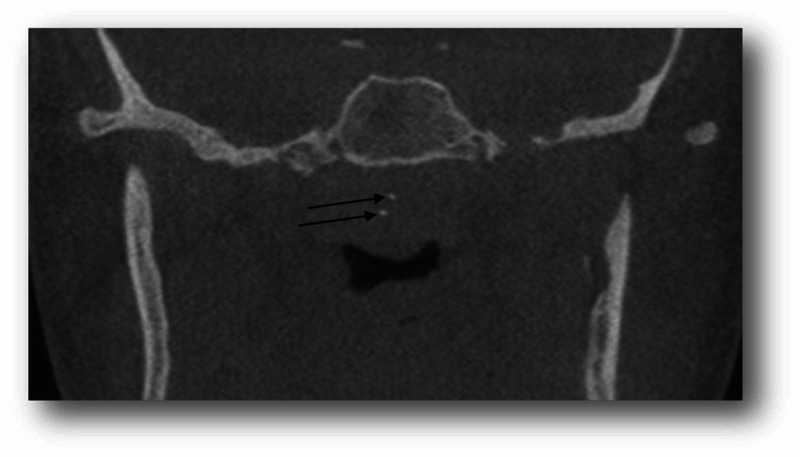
Adenoid calcifications in an 18-year-old female An 18-year-old female patient (asymptomatic) presented for dental treatment. A frontal cut of the CBCT, FOV: 8 cm (maxilla and mandible) showed multiple adenoid calcifications (black arrows) CBCT: cone-beam computed tomography; FOV: field of view
